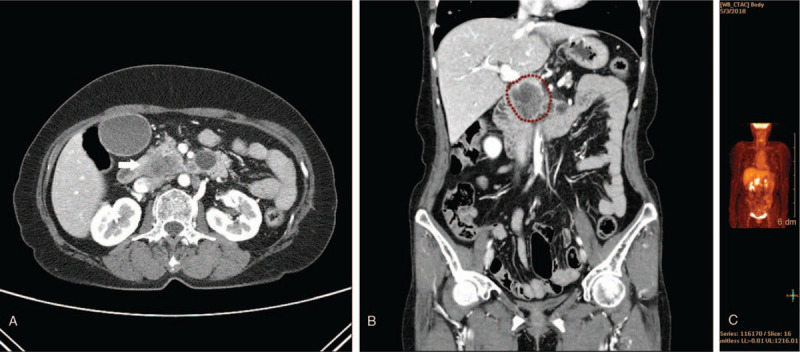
(A) Enhanced computed tomography (CT) reveals mass of uncinate process of pancreas (white arrow) with regional enlarged lymph nodes in portocaval area, porta hepatis area. Biliary tree dilation with abrupt narrowing beside the mass suggests distal common bile duct invasion. (B) A 4.2-cm-sized mass (dotted measuring line) extending vertically to portocaval area was noted. (C) Hypermetabolic lesions in both lung fields, pancreatic head, lymph nodes of porta hepatis, left paraaortic area, and sigmoid colon in positron emission tomography-CT.
